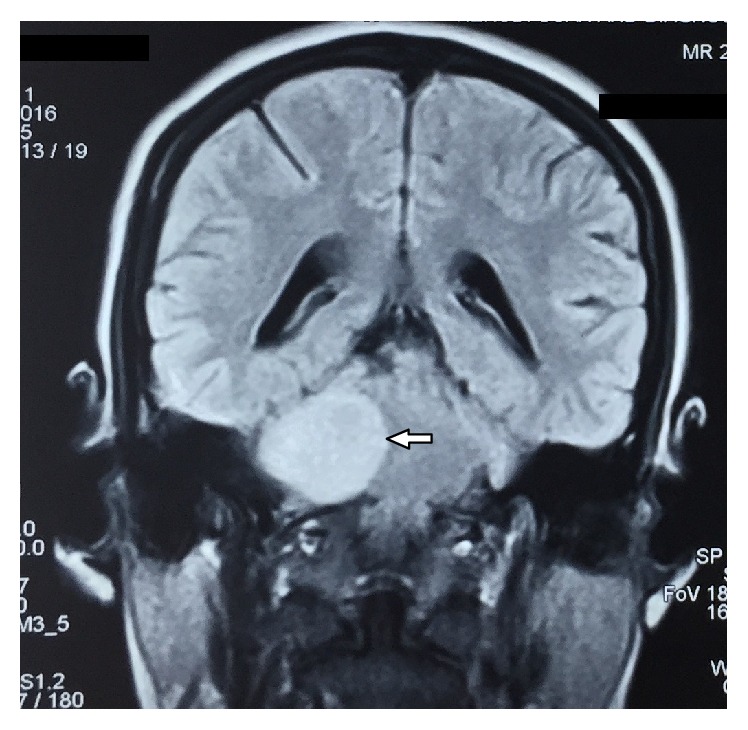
Contrast coronal MRI shows well-defined heterogeneously enhancing T1 hypo and T2 predominantly hyperintense lesion in the right cerebellopontine angle (white arrow).
